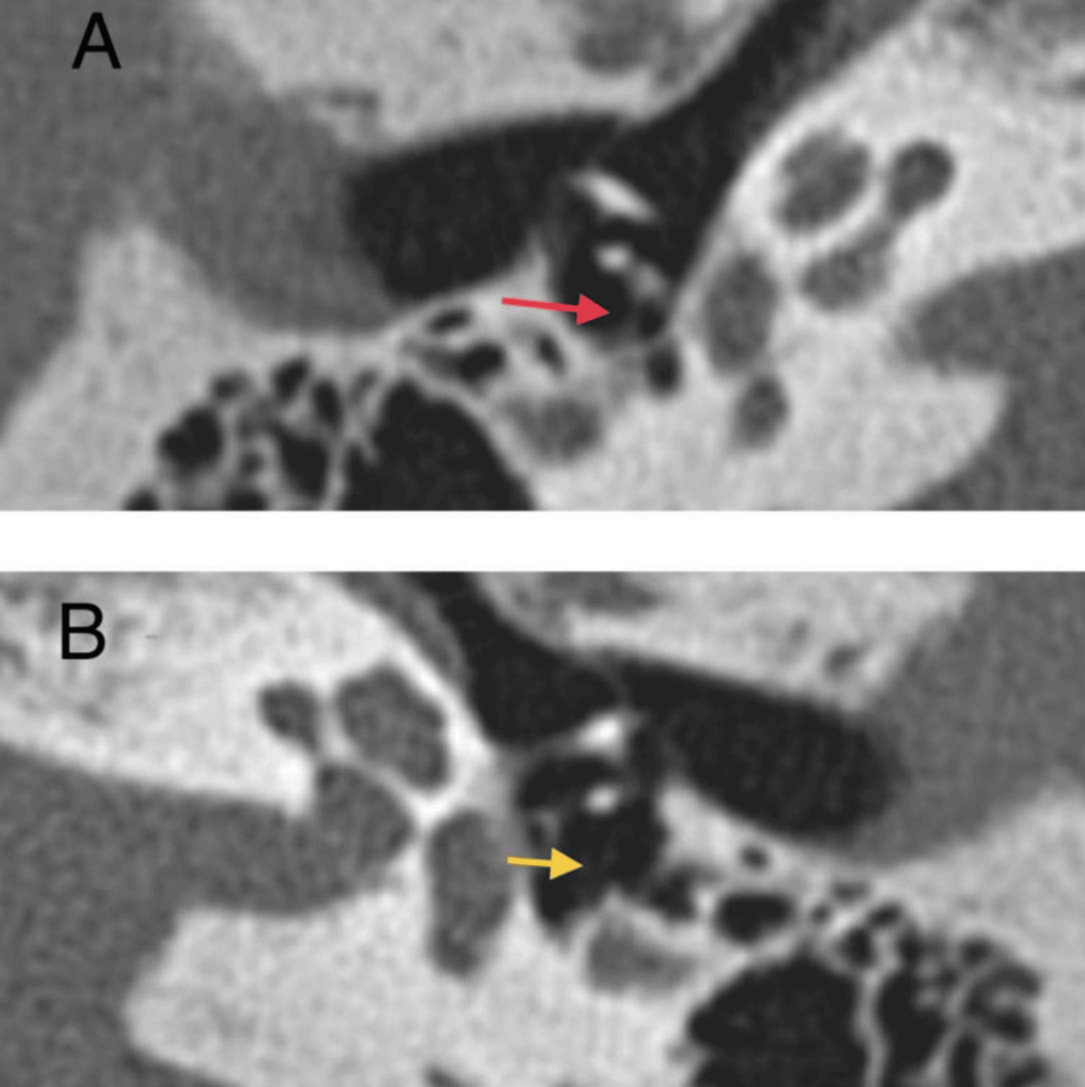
Temporal bone computed tomography (axial view). (A) The red arrow showing prominent pyramidal eminence in the right ear and the presence of a stapedial tendon. (B) The yellow arrow showing an absent stapedial tendon and non-prominent pyramidal eminence in the left ear.
